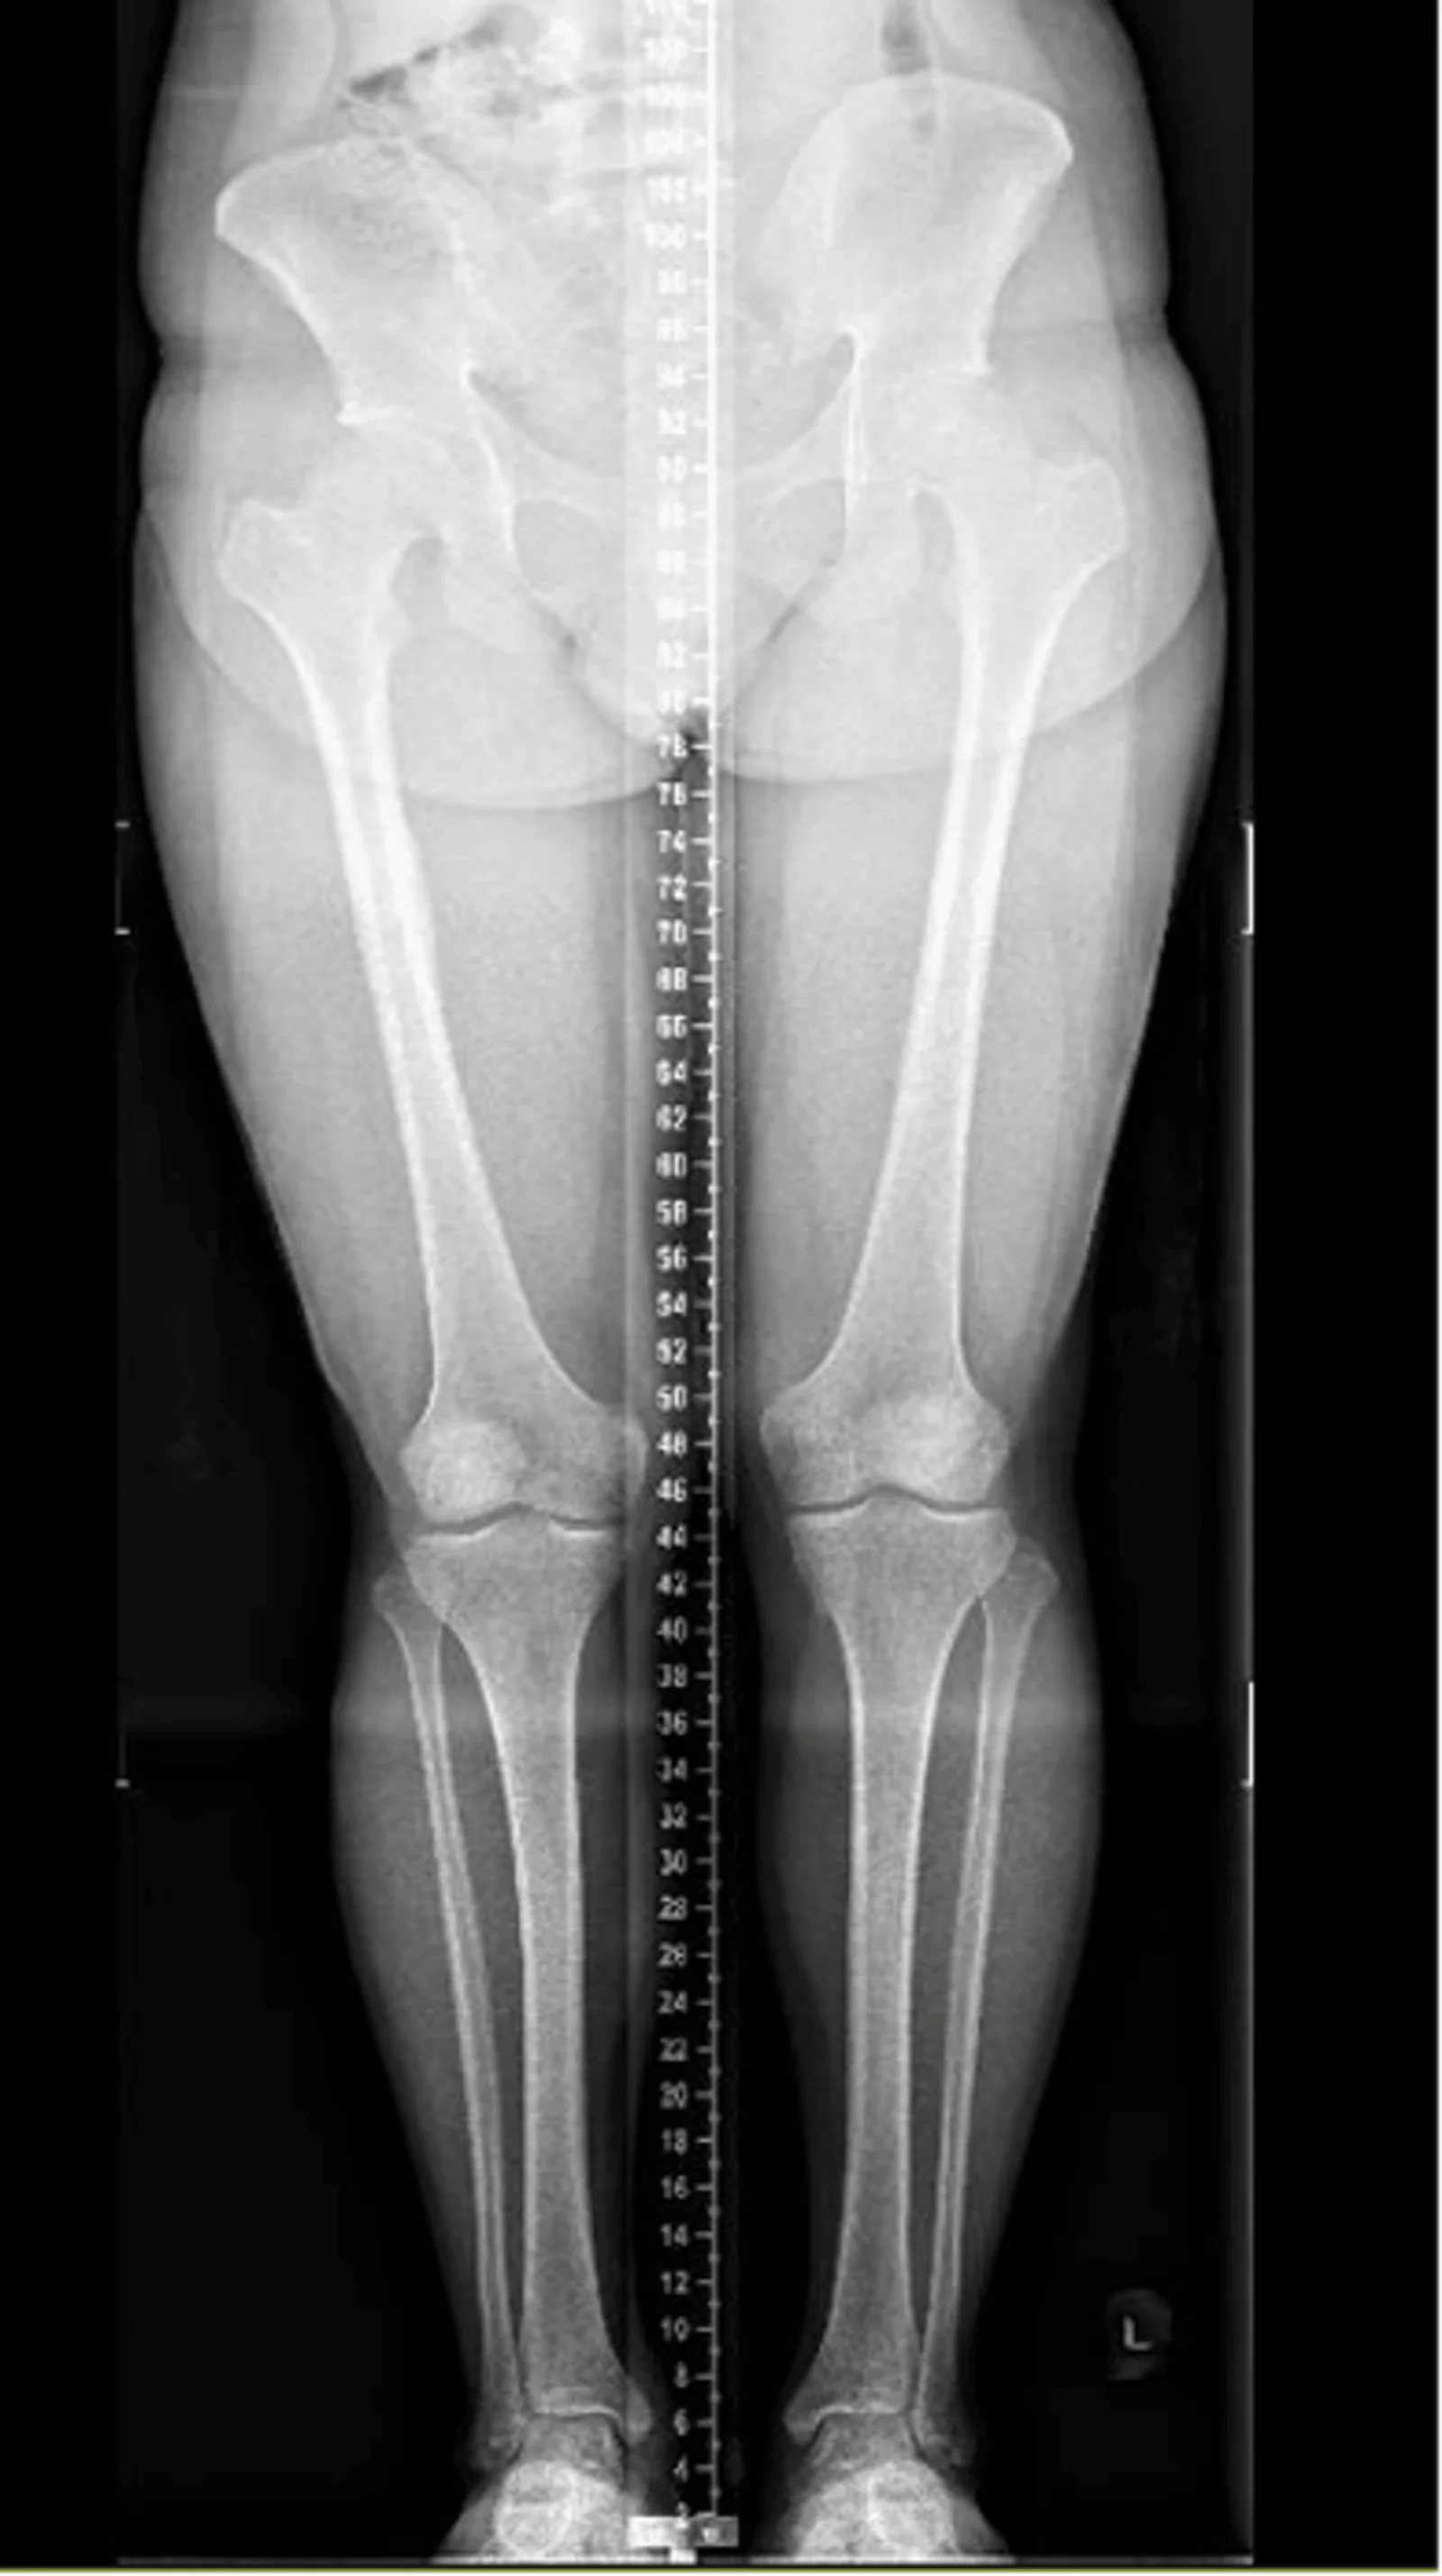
X-ray of the bilateral lower limbs showing the discrepancy in limb length.
